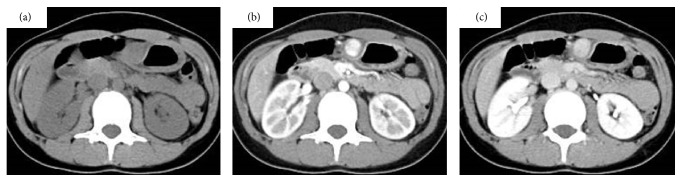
Computed tomography findings. Abdominal computed tomography revealed a well demarcated, ovoid mass at the antrum on (a) unenhanced, (b) arterial phase, and (c) delayed phase scans.
